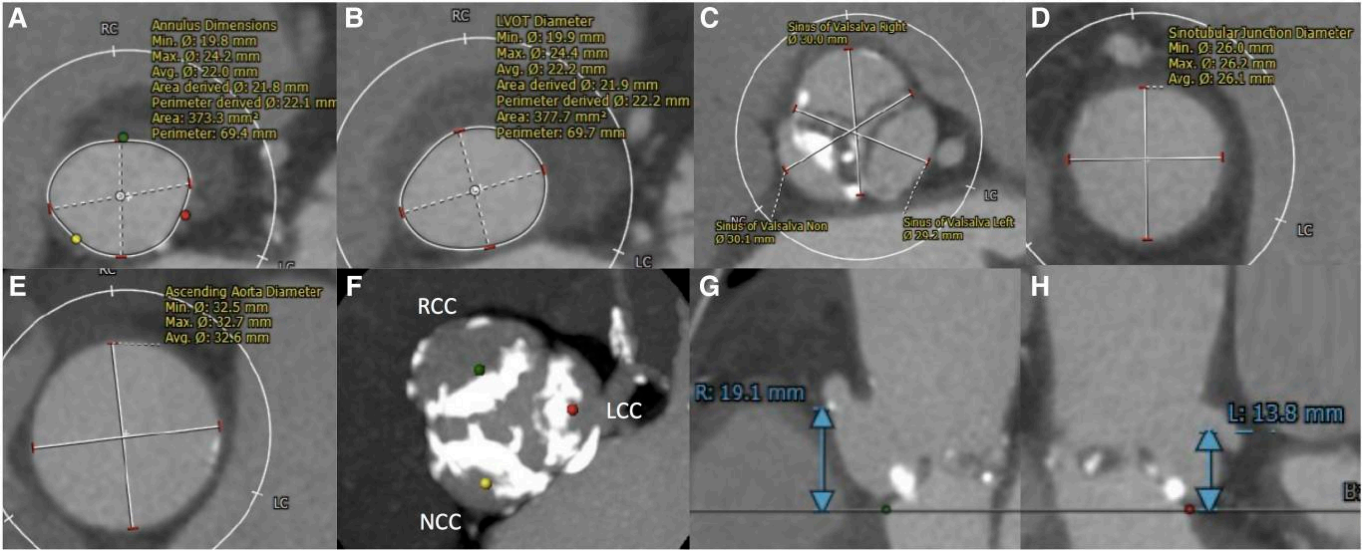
Computed tomography measurements before transcatheter aortic valve implantation. (*A*) The aortic annulus. (*B*) The left ventricular outflow tract. (*C*) The sinus of Valsalva. (*D*) The sinotubular junction. (*E*) The ascending aorta. (*F*) Leaflet calcification. (*G*) Right coronary artery height. (*H*) Left coronary artery height. LCC, left coronary cusp; NCC, non-coronary cusp; RCC, right coronary cusp.
